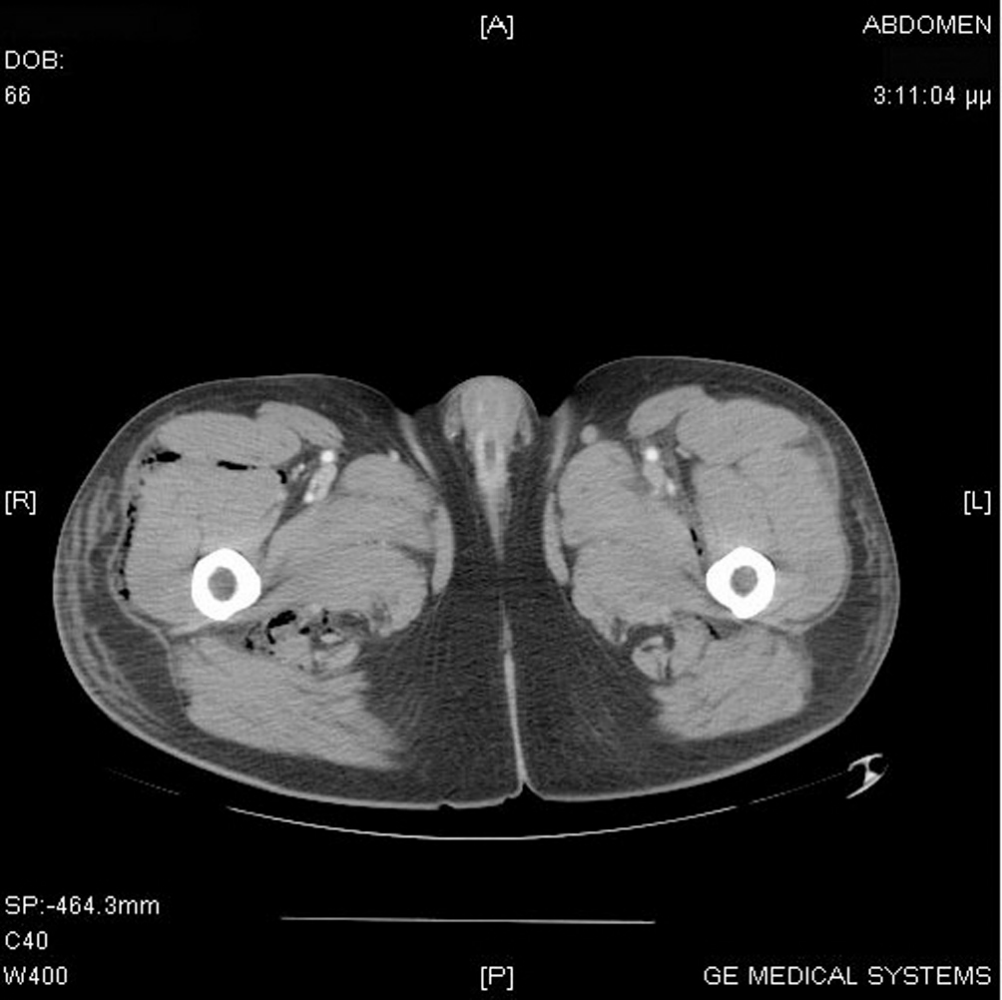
CT of the thighs revealing skin thickening, increased subcutaneous fat attenuation and subcutaneous and inter-muscular fat stranding in the right thigh.
